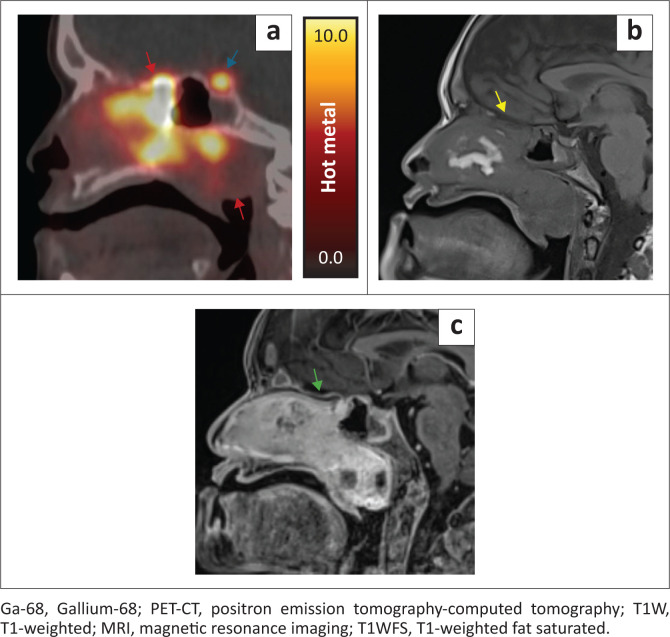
(a) Ga-68 DOTANOC PET-CT (b) T1W MRI (c) T1WFS post-contrast. Multistudy midline sagittal images through the nasal passage and nasopharynx demonstrates a mass with intense, heterogeneous DOTANOC uptake (red arrows) adjacent to an indistinct planum sphenoidale (yellow arrow) with subtle thickening and enhancement of the dura (green arrow). Physiologic pituitary DOTANOC uptake is observed (blue arrow).
